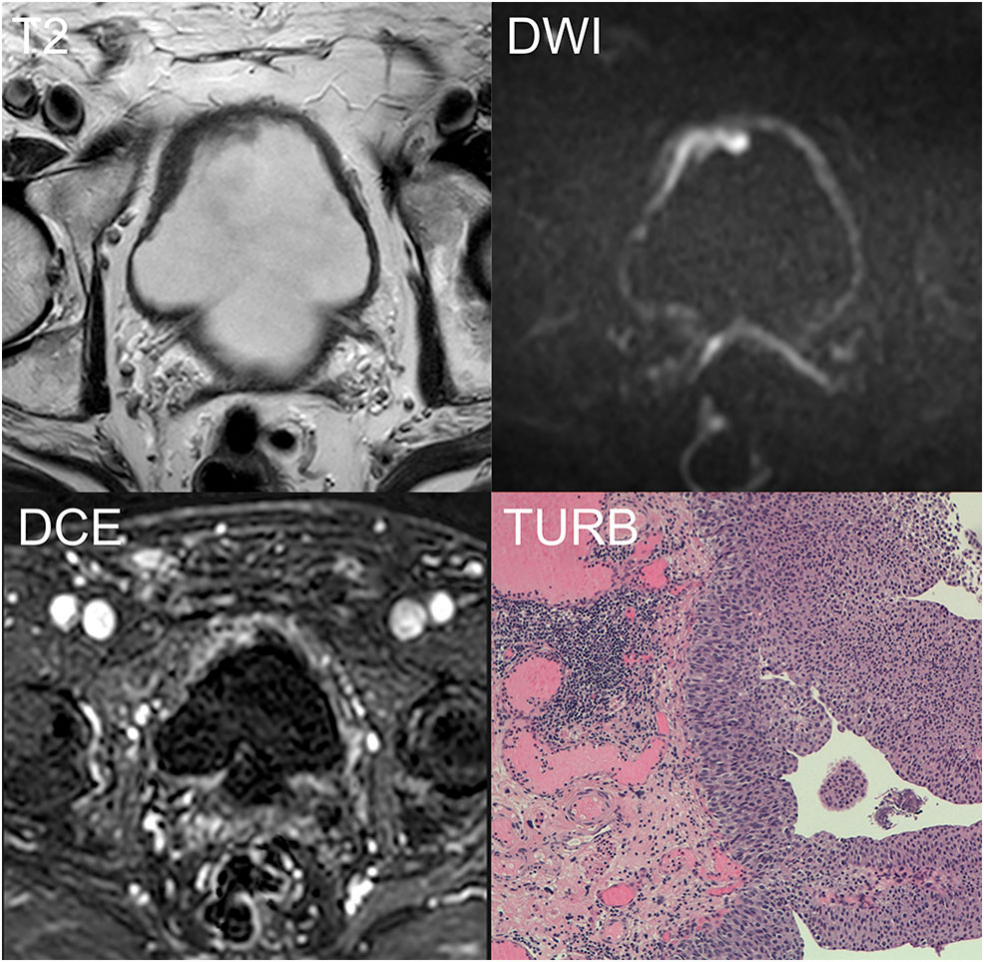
Example of incorrectly classified not muscle-invasive BC. An 89-year-old man with hematuria and bladder mass reported after flexible cystoscopy underwent mp-MRI before primary TURB. T2W imaging (T2) shows a slightly exophytic lesion on the right antero-lateral wall, which is 1 cm thick with equivocal interruption of the SI of the muscularis propria. The *b*1000-DWI (DWI) clearly demonstrated the continuous intermediate signal intensity of the muscular layer. Based on bp-MRI, three of the four readers assigned a VI-RADS category of 3. DCE imaging (DCE) showing early enhancement of the lesion with an inhomogeneous enhancement of the underlying inner layer due to inflammatory changes. This finding was misinterpreted by two readers as the muscularis propria infiltration, thus modifying the VI-RADS category from 3 to 4. T stage after TURB was LG-T1 (TURB). After 4 weeks, re-TURB was performed confirming the absence of residual tumor. DCE, dynamic contrast-enhanced; DWI, diffusion-weighted imaging; LG, low grade; mp-MRI, multiparametric magnetic resonance imaging; bp-MRI, biparametric magnetic resonance imaging; SI, signal intensity; T2W, T2-weighted; TURB, trans-urethral resection of the bladder; re-TURB, repeated trans-urethral resection of the bladder; VI-RADS, Vesical Imaging-Reporting and Data System
